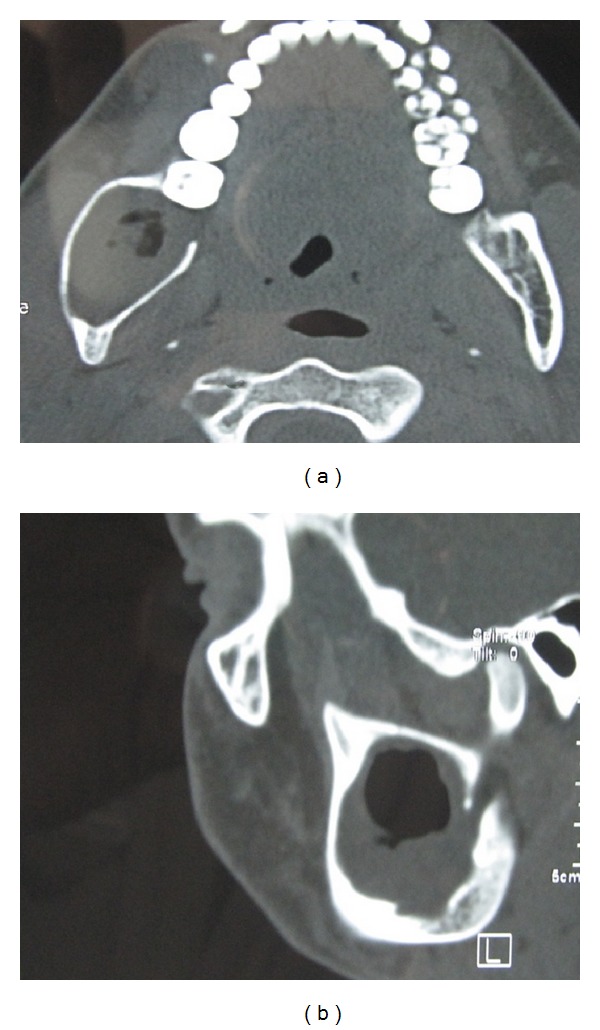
CT scan of the lesion showing a unilocular osteolytic lesion with posterior part of body and ramus of the left side of mandible revealing bilateral cortical plate expansion and perforation of the lingual cortical plate.
